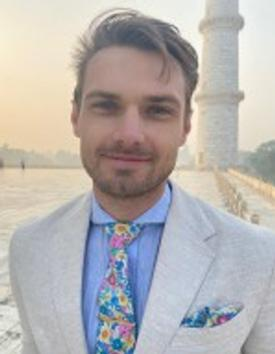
Johan O. Wedin, MD, is a resident physician and PhD candidate in Cardiothoracic Surgery at the Department of Cardiothoracic Surgery and Anesthesiology, Uppsala University Hospital, Uppsala, Sweden. He also has a bachelor’s degree in Biomedical Laboratory Science and Clinical Physiology and has previously worked as a cardiac sonographer. His research focuses on characterizing echocardiographic, surgical, and bioinformatic differences between patients with bicuspid and tricuspid aortic stenosis.
